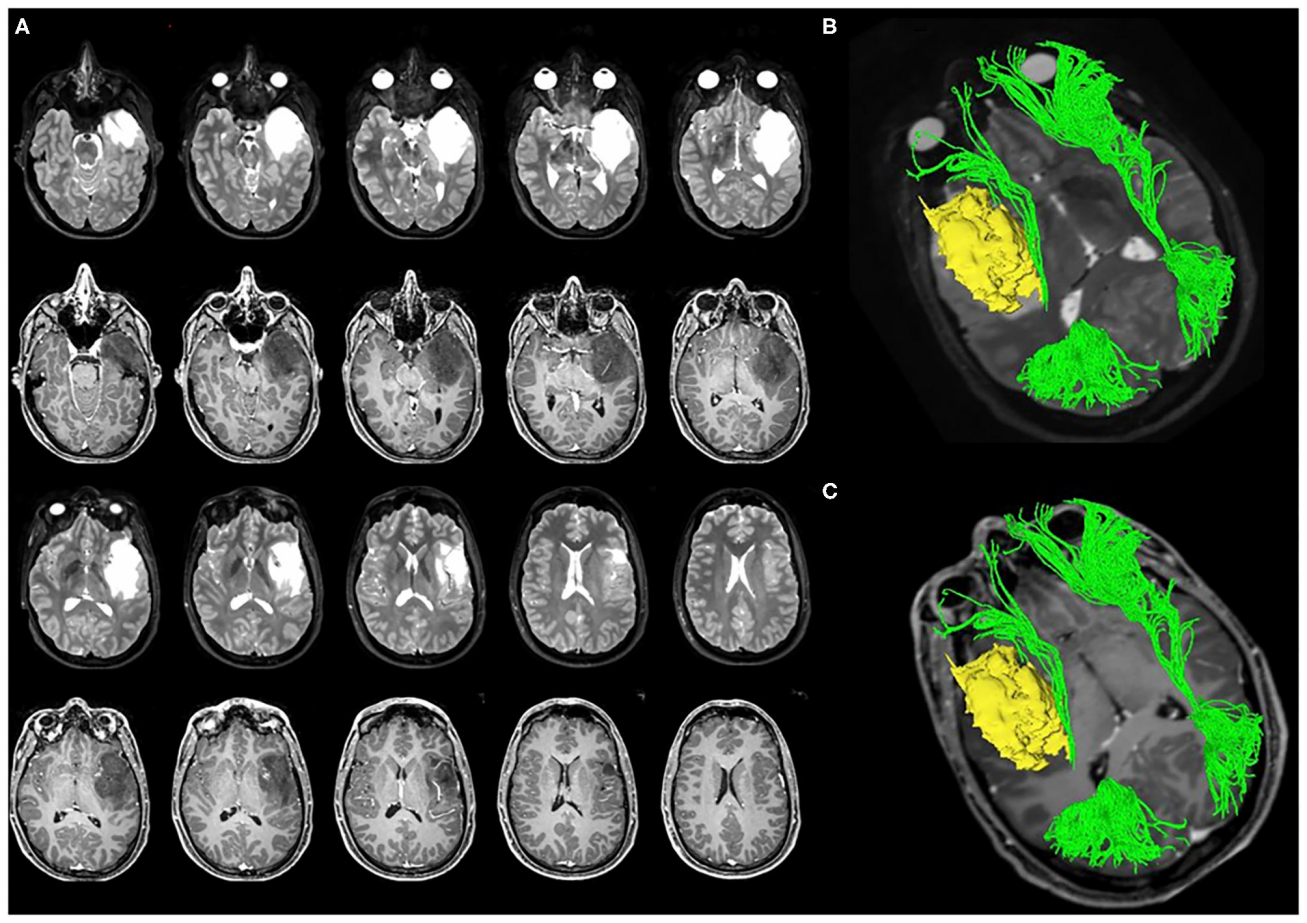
A case of left temporo-insular low-grade glioma (LGG). The pre-operative tumor volume computed on both T1-weigted MRI and on T2-weighted MRI was 38 cm^3^ (axial slices A). The tumor involves the Fronto-Occipital Longitudinal Fasciculus. (B,C) Show the 3D reconstruction of the tumor and IFOF. The pre-operative IFOF NS-index was 0.611.
